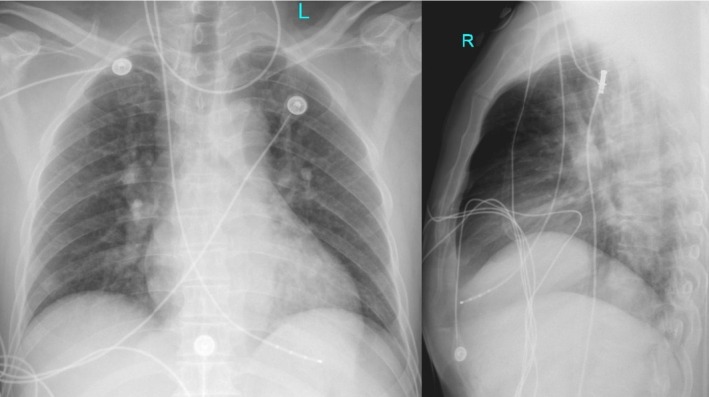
Post‐procedure chest radiograph. Posteroanterior and lateral views confirm the pacing lead positioned at the right ventricular apex.
